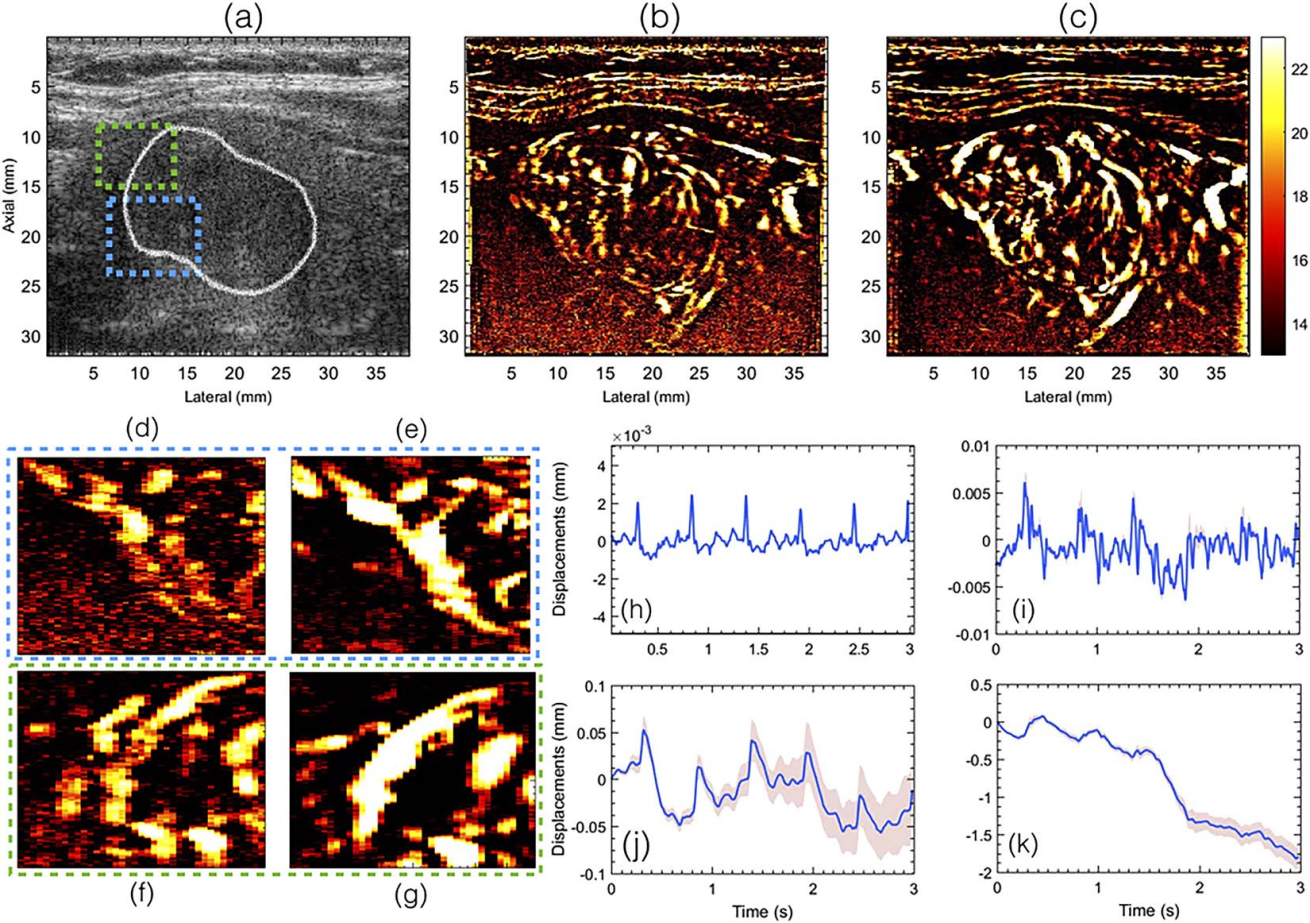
Displays the plane wave B-mode sonogram (a) and the corresponding PD images without (b) and with motion (c) correction. The outline of the thyroid nodule is indicated in white in the B-mode sonogram (a). Figures (d,f) show zoomed insets obtained from (b). Figures (e,g) show zoomed insets obtained from (c). The green and blue outline corresponds to images in (e,g) and (d,f), respectively. Figures (h,i) and (j,k) visualize the axial and lateral displacements, respectively. Further, (h,i) displays the displacements associated with every consecutive frame, and (j,k) displays the total accumulated displacements, with reference to the first frame in the ensemble. The continuous error-band (red) displays ±1 standard deviation from the mean.
